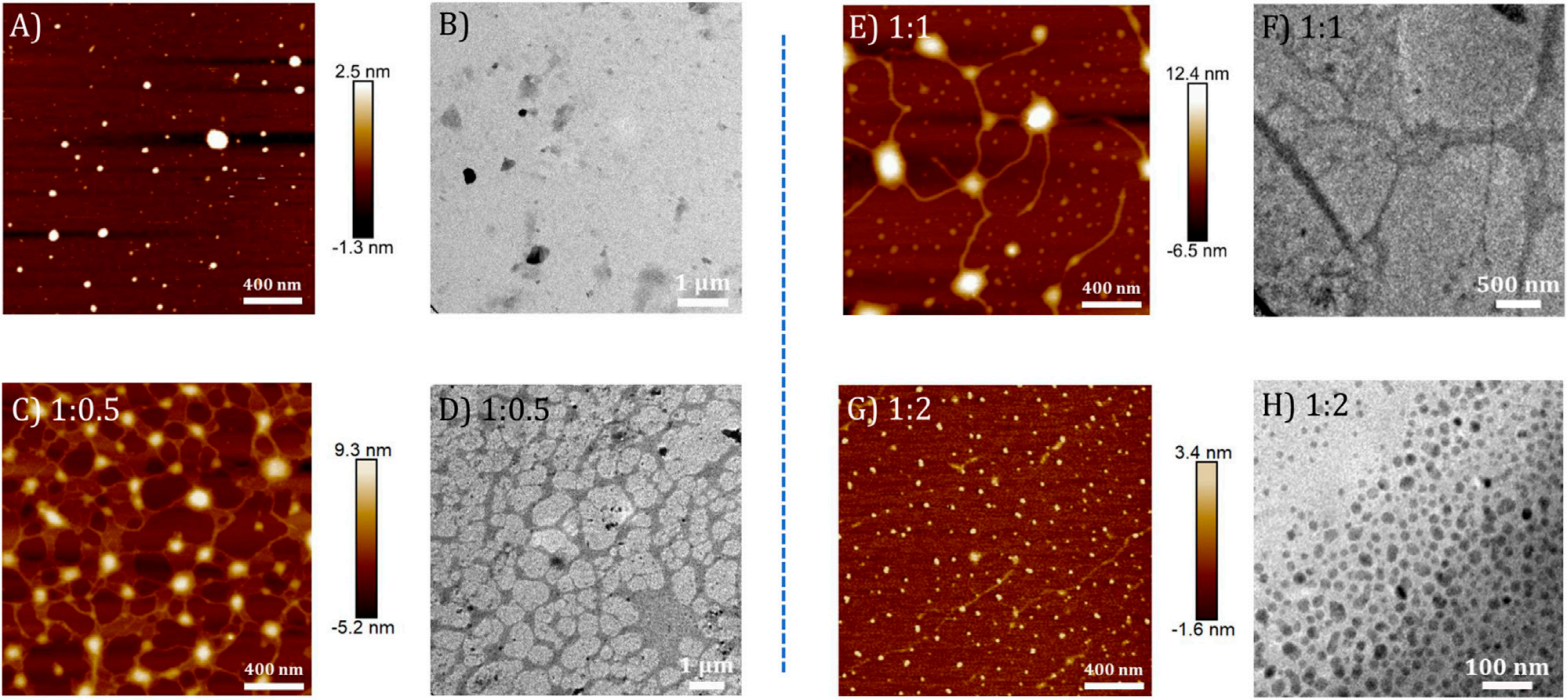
AFM and corresponding TEM images of (A,B) TPE-V2 (10 μM) and TPE-V2/CT-DNA complex at different CT-DNA to TPE-V2 ratios: (C,D) 1:0.5, (E,F) 1:1, and (G,H) 1:2.
